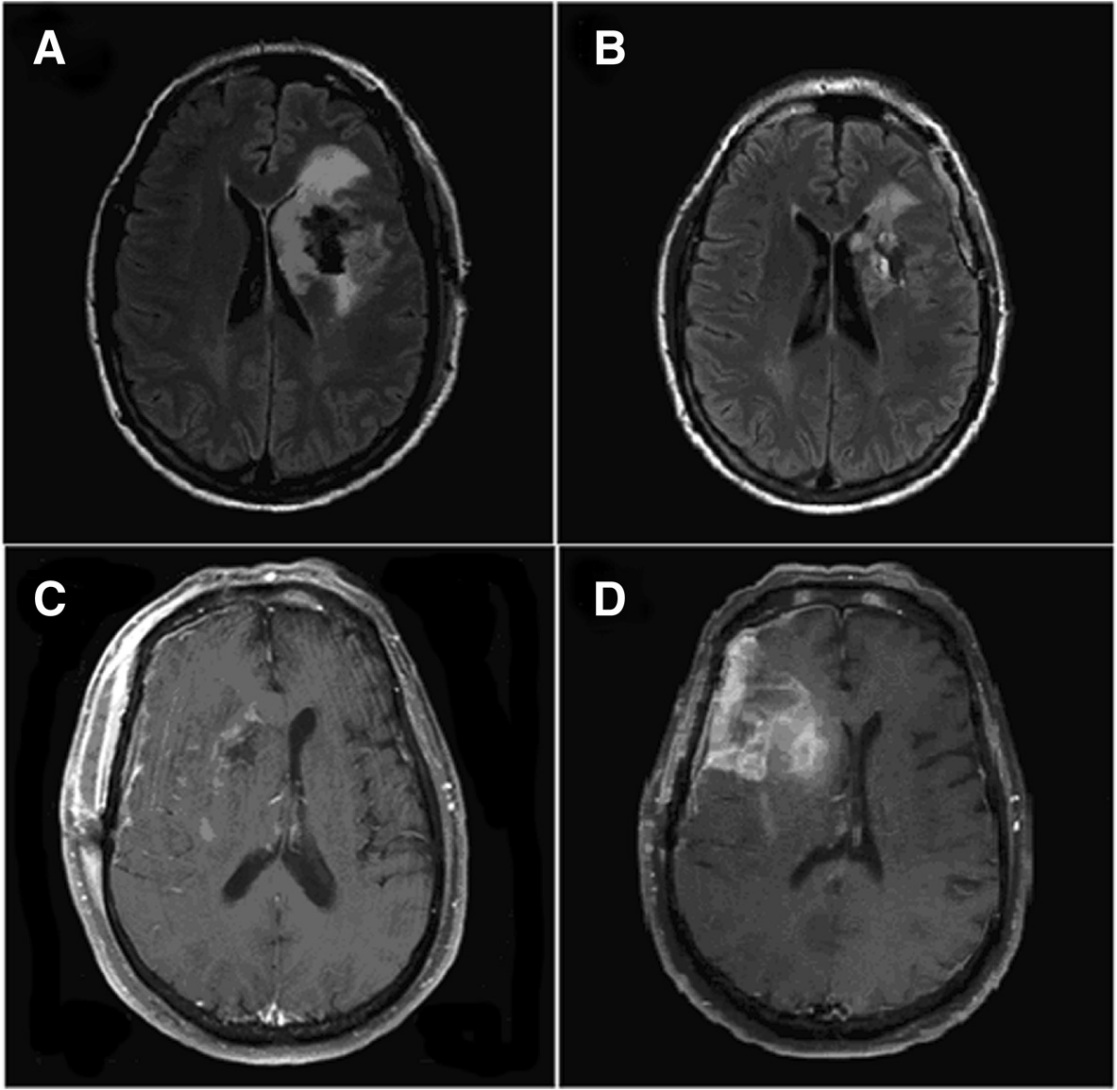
Changes in volume on T2/FLAIR and T1 contrast-enhanced MRI: A. T2/FLAIR MRI performed 1 day after craniotomy. B. T2/FLAIR MRI performed 2 weeks after craniotomy. Another patient with C. T1 contrast-enhanced MRI performed 1 day after craniotomy. D. T1 contrast-enhanced MRI performed 2 weeks after craniotomy.
